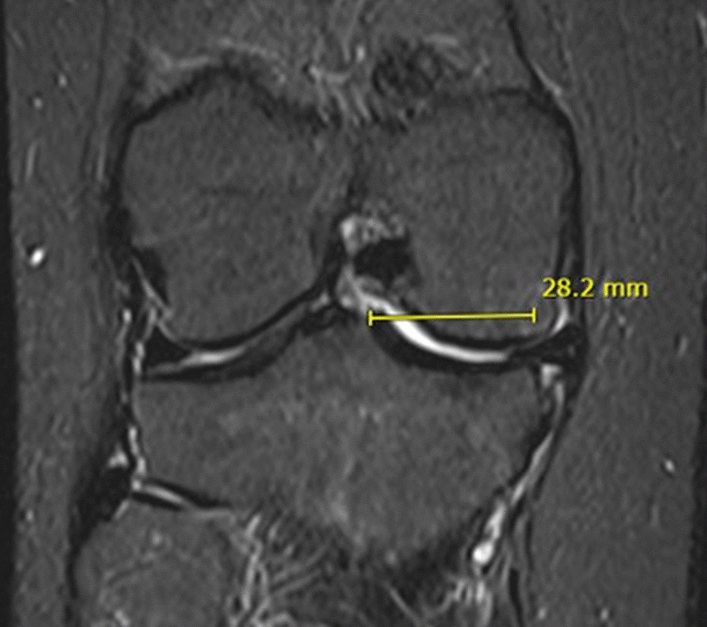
Medial tibial plateau width on Coronal MRI
